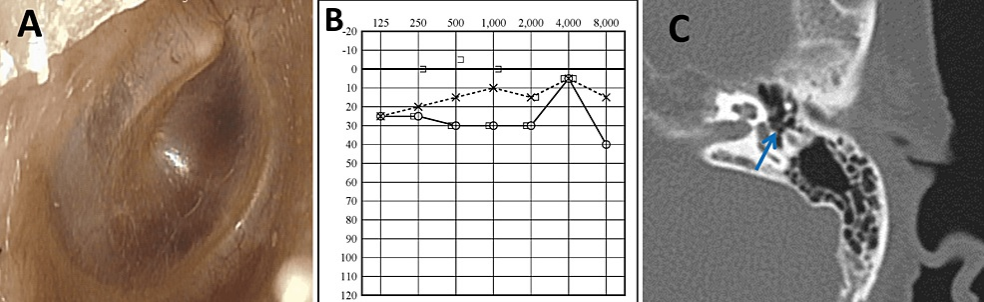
Ear findings after four months of treatment. (A) Otoscopic findings after treatment. The tympanic effusion resolved. (B) Pure tone audiometry after treatment. The air-bone gap is reduced in both ears. (C) Temporal bone CT scan. After treatment, soft tissue shadows in the bilateral tympanic cavities lightened (blue arrow).
